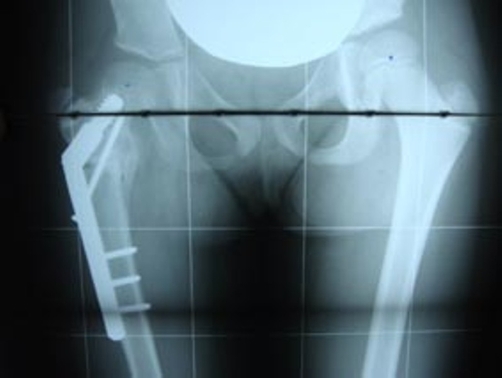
Anteroposterior pelvis radiograph demonstrates osteotomy healed.
